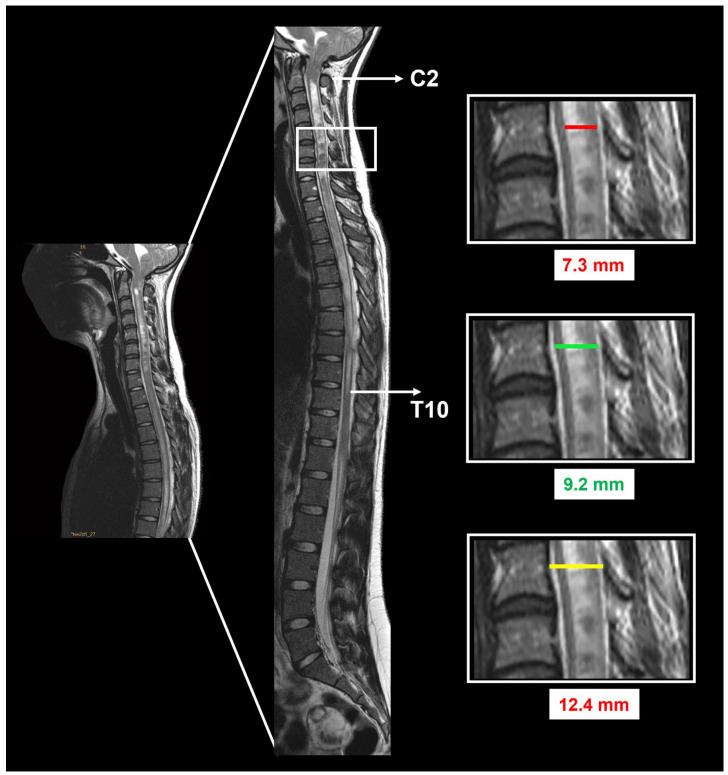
Calculation of the extension and diameter of the syrinx cavity. The extension of the syringomyelia (Syr) was quantified as the number of vertebrae between the superior and inferior limits of the cavity (in this patient, between C2 and T10). To calculate the syringo-cord ratio (SCR) and the syrinx–canal index (SCI), we measured the Syr maximum anteroposterior (AP) diameter at its widest level on the midsagittal MRI (red line, 7.3 mm), the maximum AP diameter of the spinal cord at the same level (green line, 9.2 mm), and the spinal canal diameter (yellow line, 12.4 mm) at the same level at which the maximum AP diameter of the syrinx was measured (white frame). The SCR was calculated by dividing the syrinx’s maximum AP distance by the spinal cord’s AP (SCR %: AP diameter of the syrinx/AP diameter of the spinal cord × 100 = 79.3%). The SCI was calculated by dividing the syrinx’s maximum AP distance by the spinal canal’s AP at the same level (SCI %: AP diameter of the syrinx/AP diameter of the spinal canal × 100 = 58.9%).
